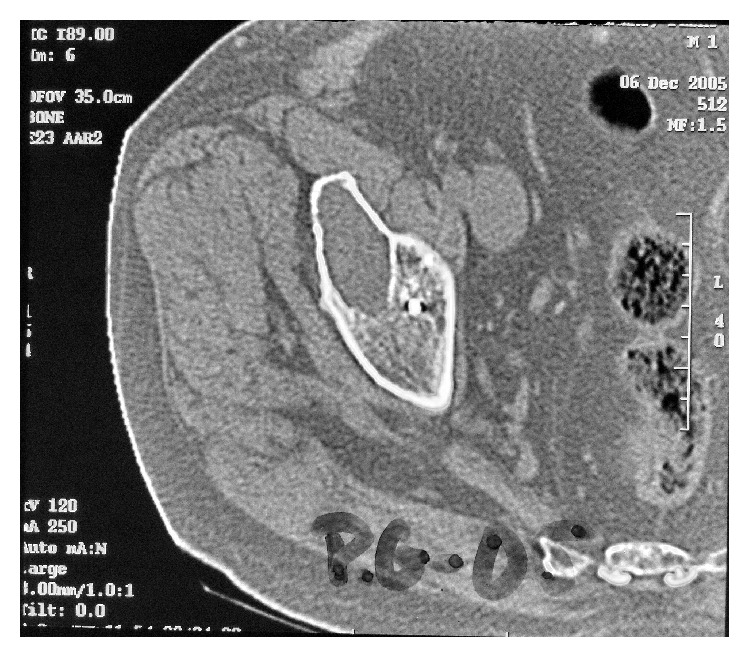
Computed tomography of the same case in Figure 2. Osteolysis in acetabulum.
